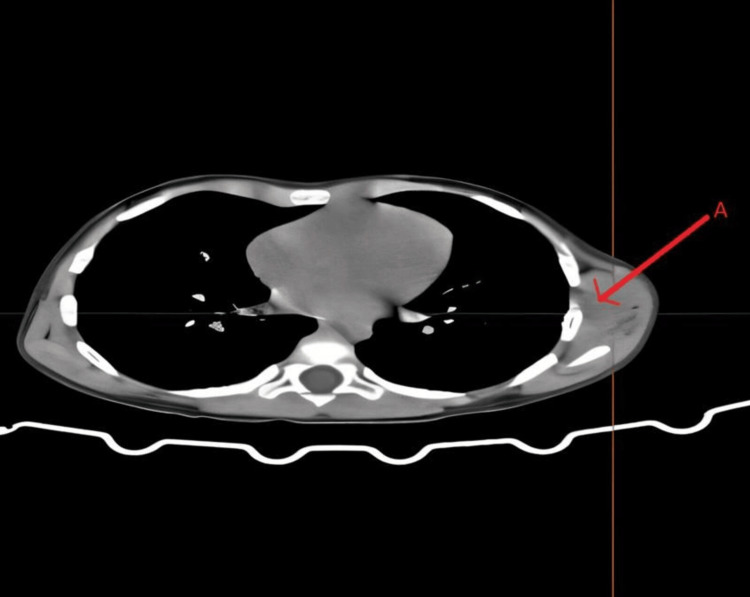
CT image showing a benign mesenchymal lesion, likely a hemangioma (A) A well-defined, ovoid mass lesion isodense to muscle is seen in the left posterolateral chest wall (red arrow), deep to the serratus anterior and overlying the costal muscles. The lesion shows no obvious invasion of the adjacent ribs or pleura. The overlying muscles show normal density.
